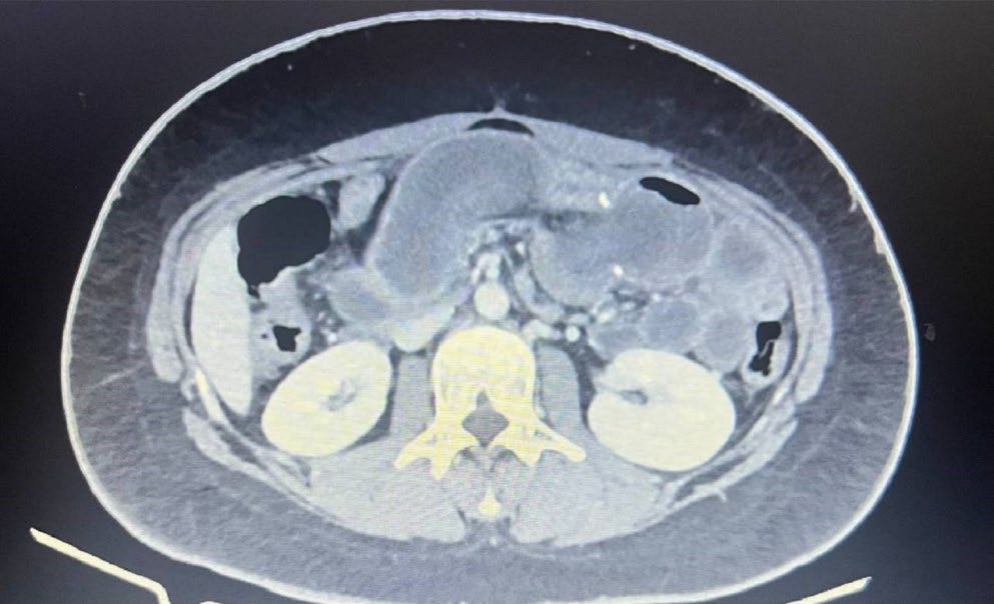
Axial abdominal CT demonstrating the classic target/bull's‐eye sign.
